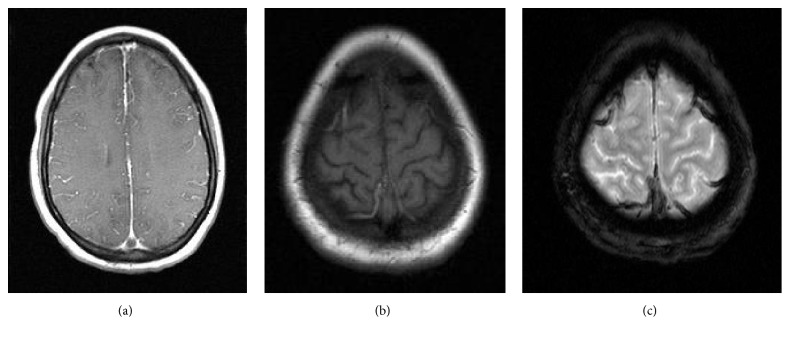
MRI brain with and without contrast showed the MRI equivalent of the “empty delta sign” (a) on postcontrast T1 images. Note the hyperintense cortical veins on T1 (b), which correspond with hemosiderin deposits on GRE (c).
